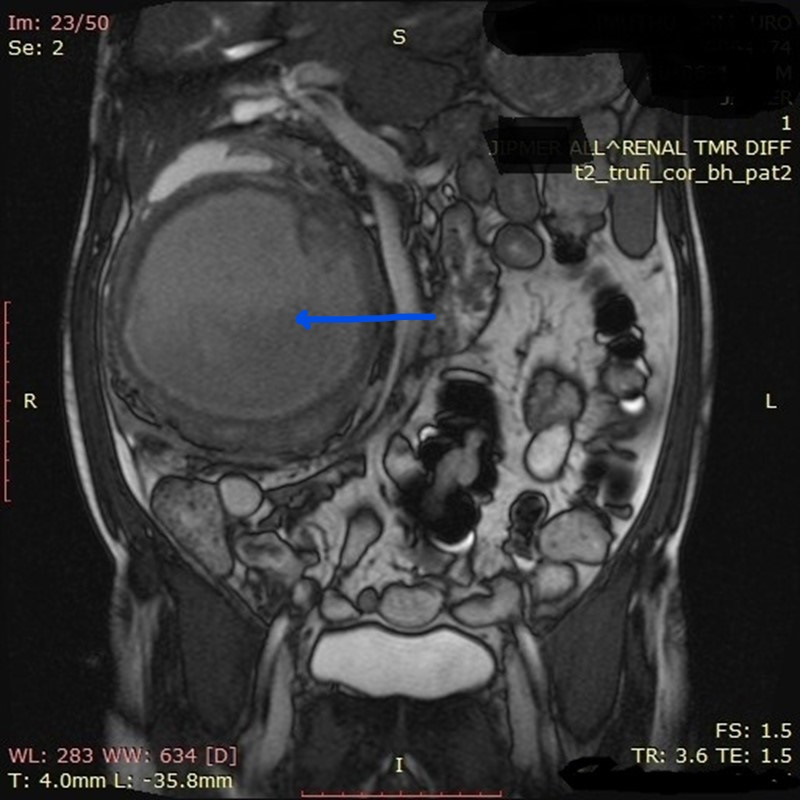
MRI of the abdomen and pelvis showing heterogenous hypointense large right renal mass, arising from lower pole, fat plane maintained with liver, IVC compressed by mass and pushed laterally.
